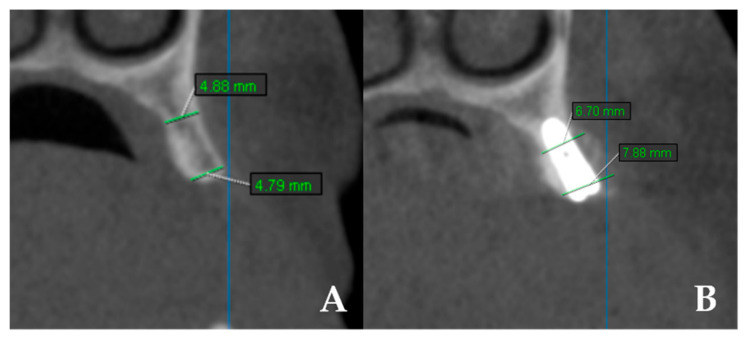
CBCT images showing linear measurements of ridge width before and after surgery. (A) Preoperative measurements of horizontal ridge width. (B) Postoperative measurements of horizontal ridge width.
